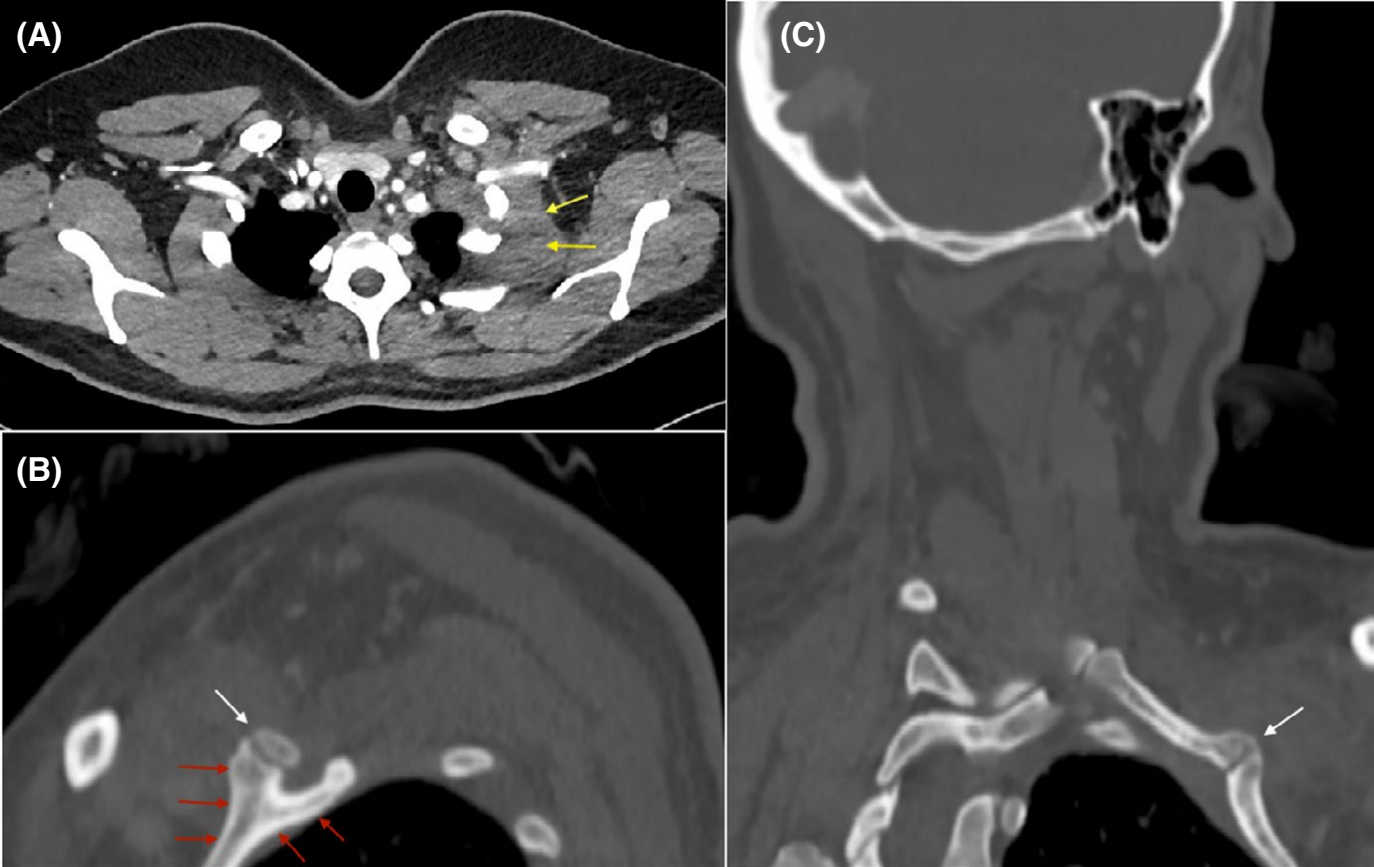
CT chest. (A) The axial CT image at the apices’ level demonstrates thickening of the left scalene muscle with associated soft tissue thickening/hematoma around the left cervical rib's pseudoarthrosis with the first rib (yellow arrows). (B) Sagittal and (C) oblique coronal CT images of the left side of the chest show deformity of the left first rib with bifid appearance anteriorly (red arrows) and associated pseudoarthrosis with the anterior end of the cervical rib (white arrow)
